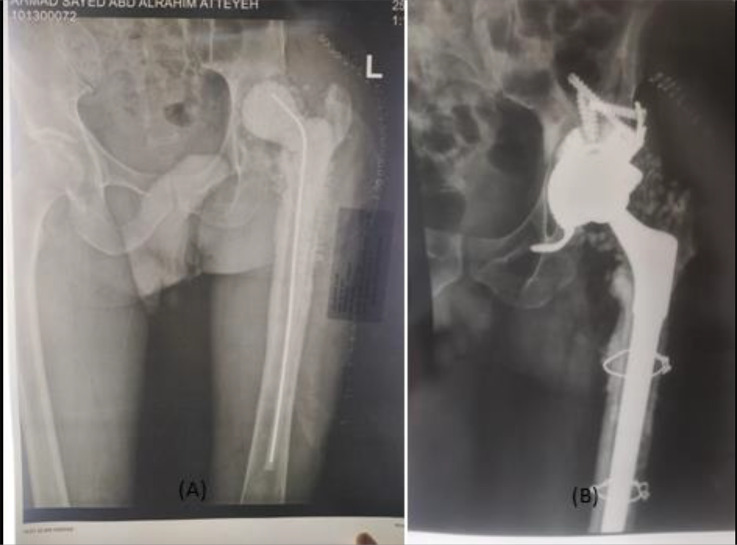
(A) preoperative X-ray for a case with hip spacer and acetabular defect, (B) postoperative X-ray after reconstruction using Delta TT revision system.
